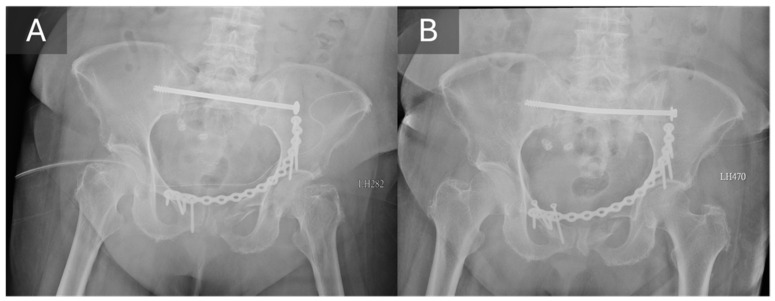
The case with mechanical failure requiring revision surgery in the present study. Though good alignment shown on the radiograph immediate after operation (A), TITS screw loosening with back-out and bending was revealed by the 1-month follow-up radiograph (B). The back-out of the anterior fixation also indicated instability. Due to the mechanical failure and the persisted pain, the patient underwent a revision surgery.
